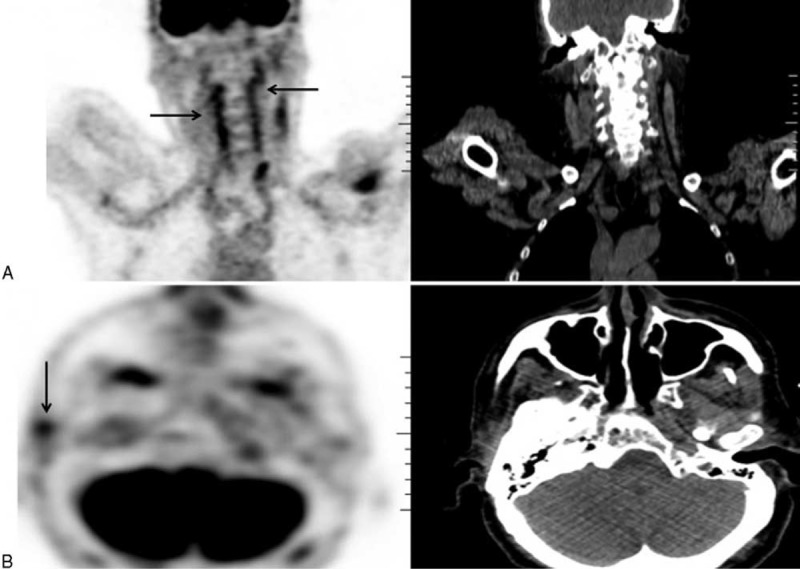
Coronal PET with CT scan. Intense 18-FDG uptake of both vertebral arteries (A) and external carotid arteries branches including temporal artery (B).
